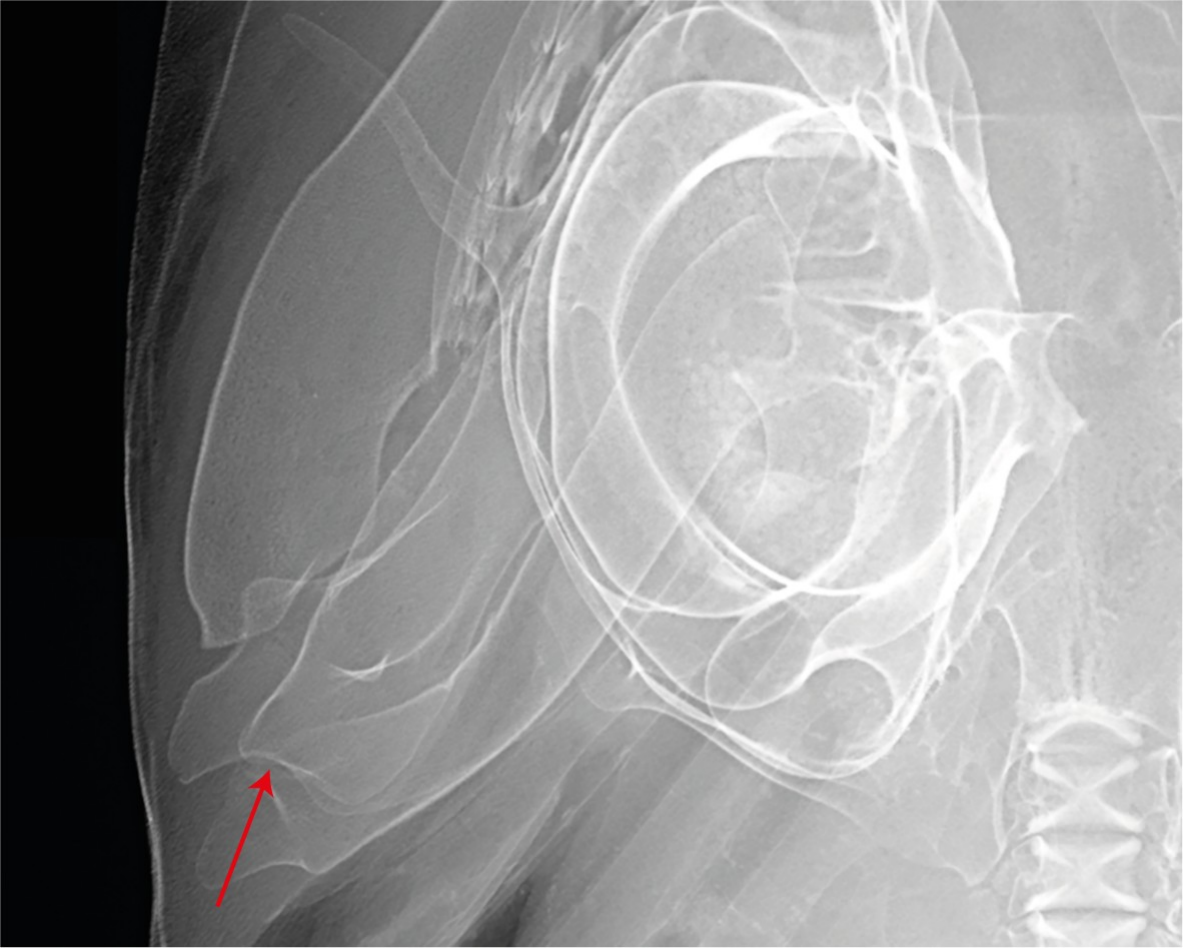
Post-mandibular indentation of Meckel’s cartilage in *Carcharhinus obsolerus* sp. nov. Radiograph of head of ANSP 77121, paratype; 433 mm TL female; arrow denotes indentation.
